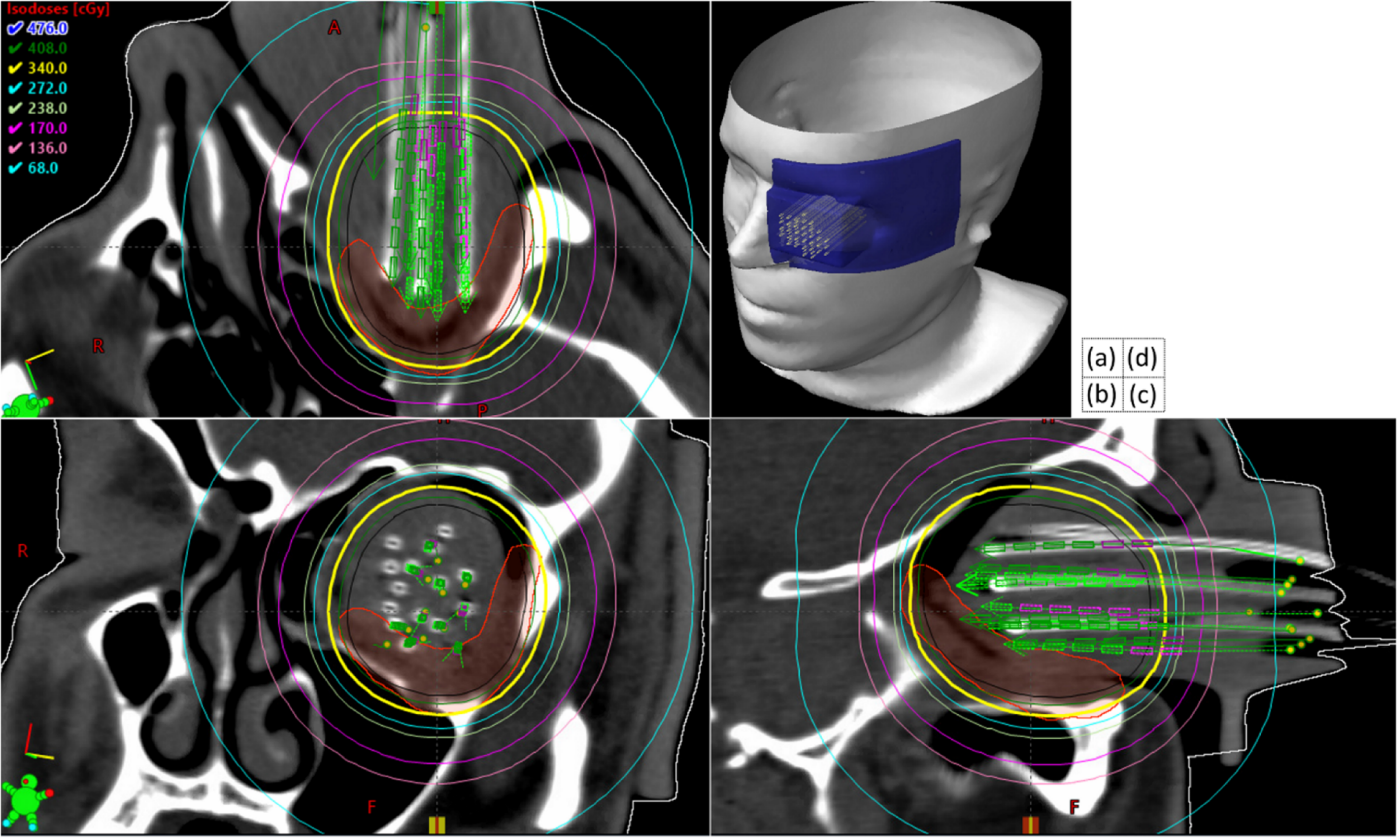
CT scan of the applicator immobilized in the orbit in (a) axial, (b) coronal, (c) sagittal, and (d) model view. Patient surface, PTV, and applicator are represented in white, red, and blue, respectively. Note that this CT scan contains the custom applicator in the patient’s orbit. Isodose lines are shown for a prescription of 340 cGy (yellow line)
